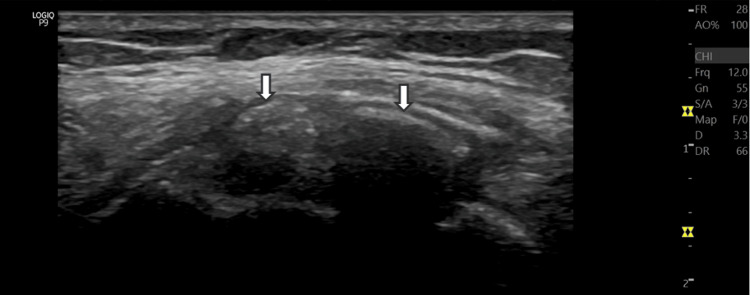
Ultrasound image Longitudinal ultrasound image showing focal calcification foci (arrows) within the proximal fibers of the LCL LCL: lateral collateral ligament
